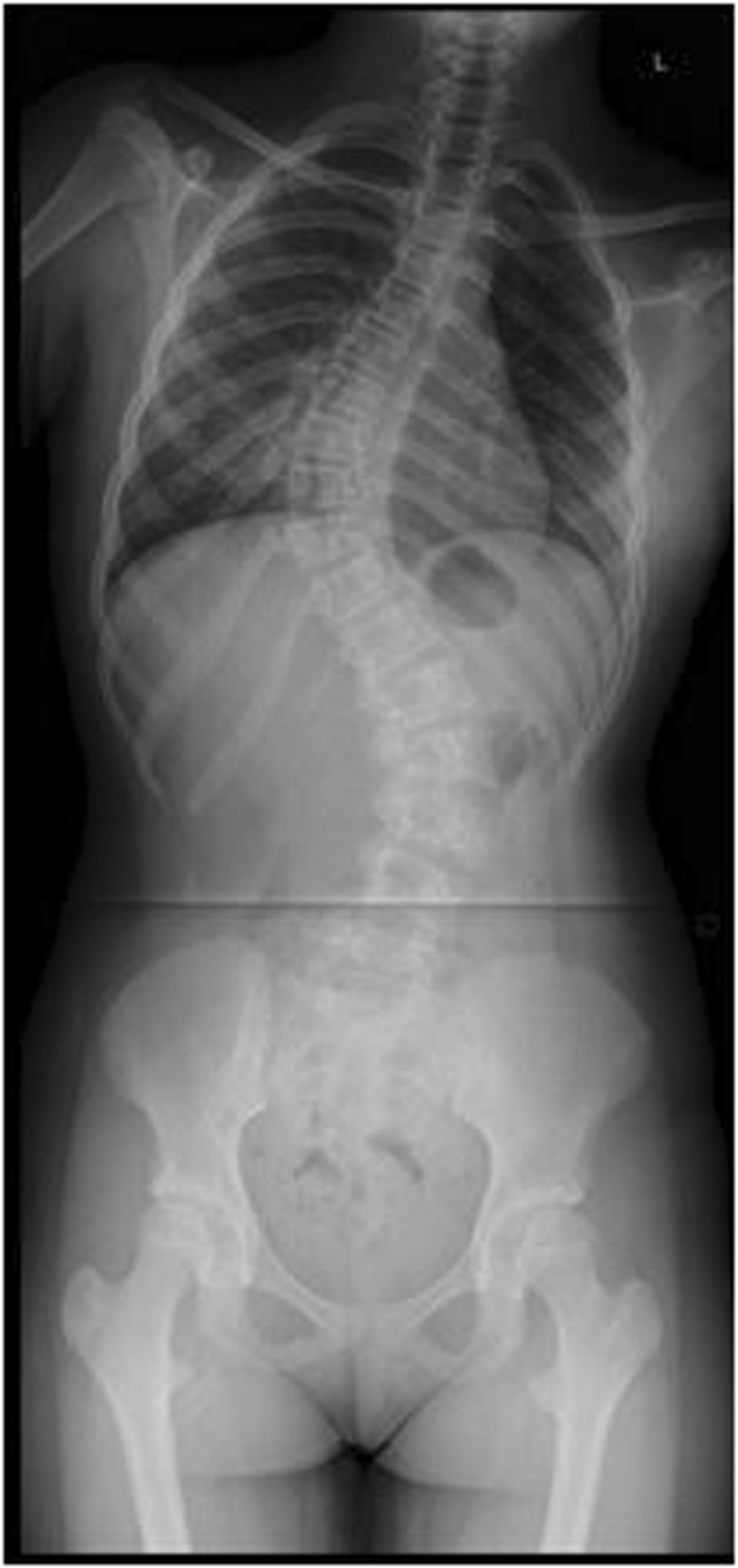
Preoperative radiograph of 12 year-old female with Down syndrome.
